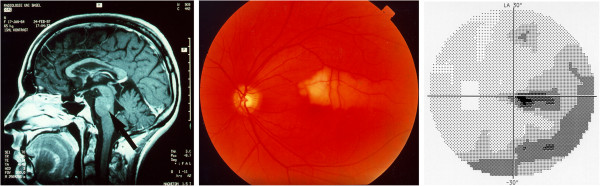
Susac syndrome in a patient with severe PVD. (Left) MRI of the pons with a hyperintensive lesion. (Middle) Localised retinal infarction. (Right) Corresponding visual field defects. (From [271], with permission).
